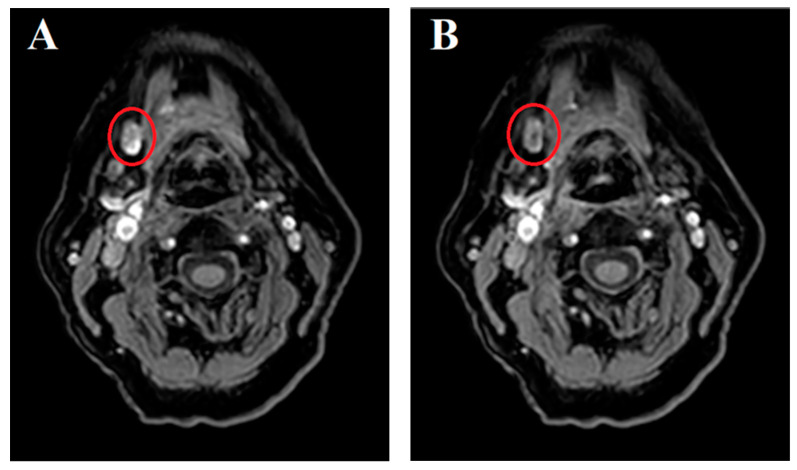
Dynamic MR lymphography. Contrast-enhanced dynamic MR images acquired for 10 min after peritumoral injection of undiluted gadobutrol in the same patient as Figure 1 (patient 2; Table 2). Rapid lymphatic drainage of gadobutrol was observed through dynamic MR lymphography, with gadobutrol washed out from the SLN within 7 min. (A) Initial dynamic MR depicting the sentinel lymph node in level Ib on the right side (red circle). (B) MR imaging acquired at 6 min and 23 s following (A), showing the same SLN in level Ib on the right side (red circle).
